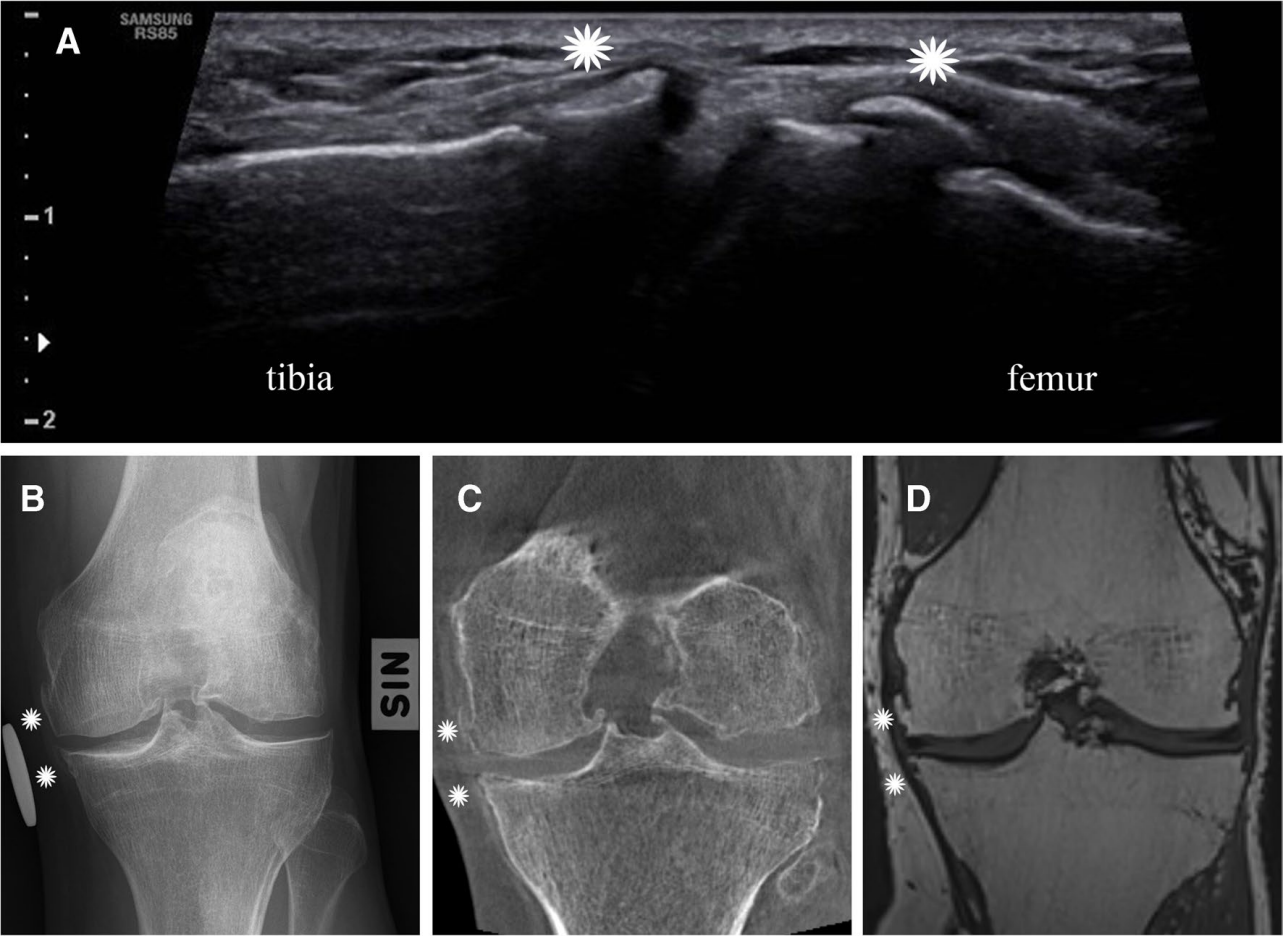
A 54-year-old male with medial osteophytes (white asterisks) of the knee joint as depicted by longitudinal US (A), anteroposterior CR (B), coronal CT (C), and coronal T1-weighted MRI plane (D). In general, all the modalities provide good visualization of the osteophytes (white asterisks). However, based on the multiple studies reviewed here, the US seems to be superior to CR for detection of osteophytes
